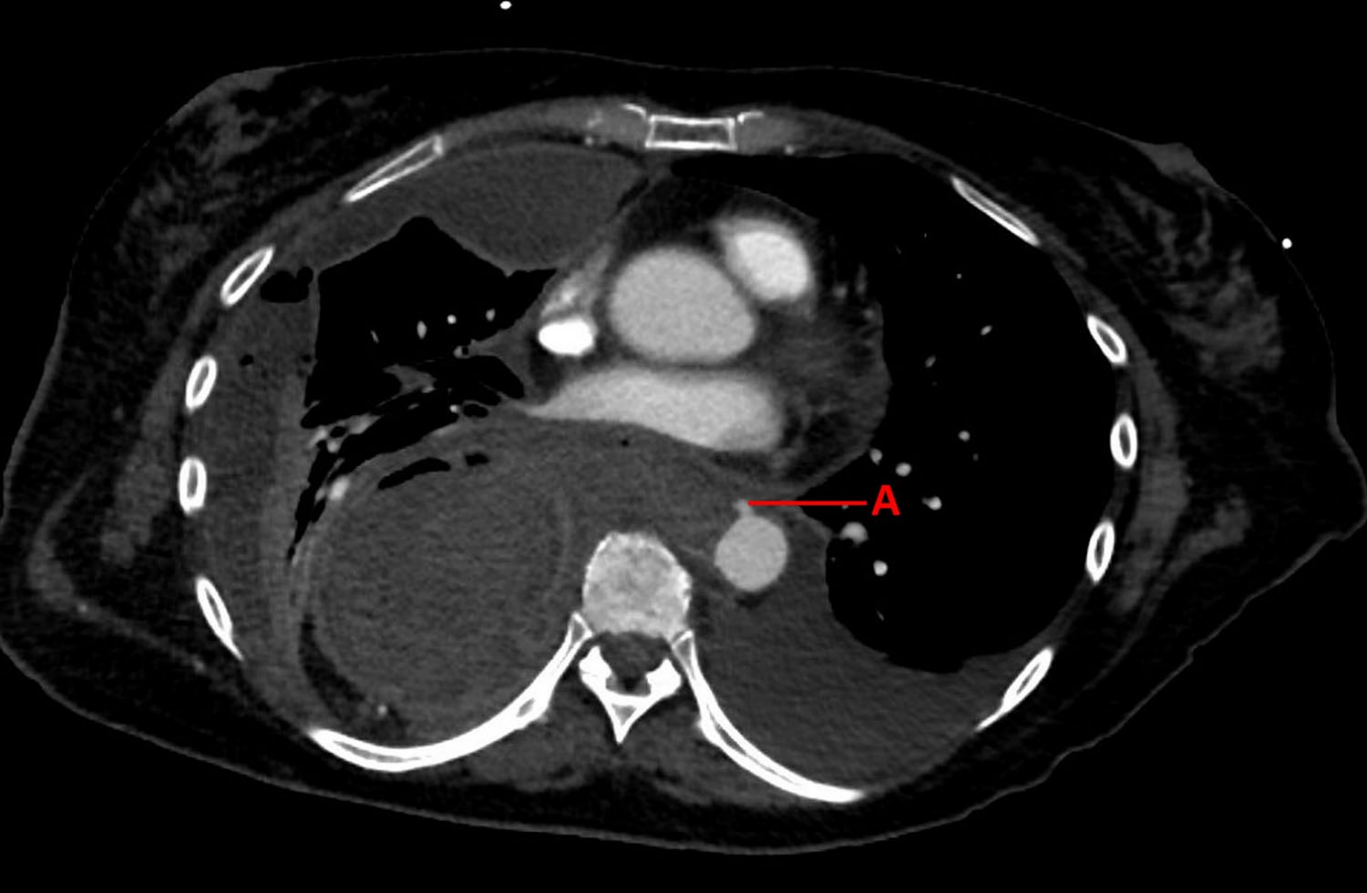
CT angiography with aortic blush (A) adjacent to the intrathoracic anastomosis
